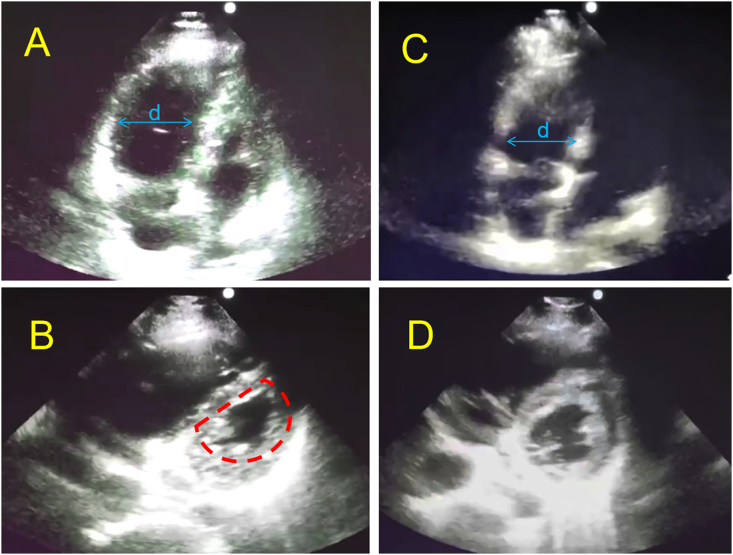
The cardiac point-of-care ultrasound of patient 1 before and after thrombolytic therapy. A: before thrombolytic therapy, apical five-chamber view: right ventricular significantly enlarged, RV: LV>1; B: before thrombolytic therapy, parasternal short axis， classic D shape sign, more obvious during systolic phase； C: after thrombolytic therapy (T + 0:70), RV size became smaller, RV: LV < 1, D: after thrombolytic therapy, IV septum deviation improved; D: shape sign disappeared.
